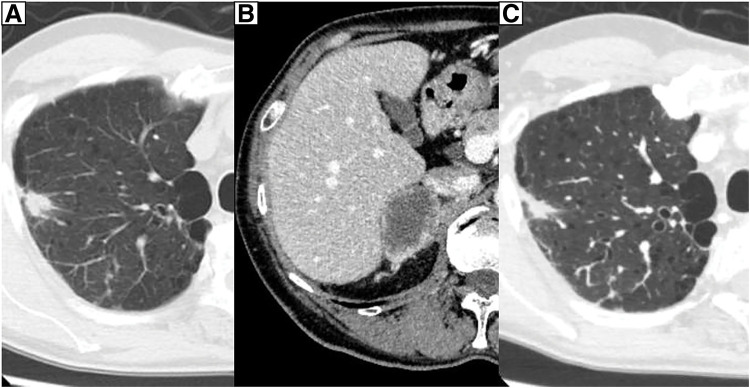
Contrast-enhanced CT of the thorax and abdomen. CT shows an irregular 2-cm-sized nodular shadow with spicules just below the dorsal segment (S2) pleura of the right upper lobe (A), and a 4.5-cm mass was observed in the right adrenal gland (B). On CT following adrenalectomy, the perpendicular diameter of the primary lesion had decreased to 1.0 cm (C).
